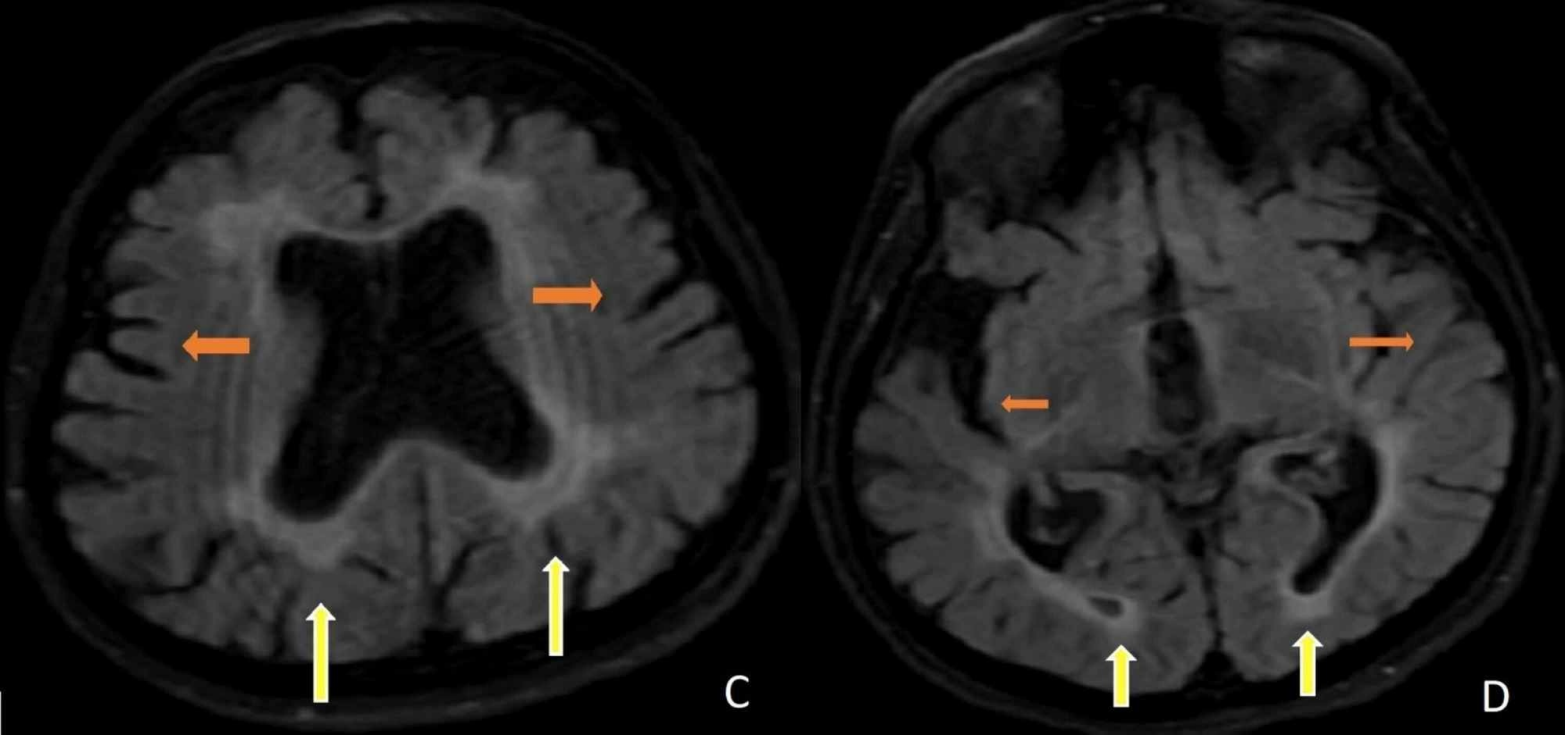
C-D. Axial FLAIR brain MRIs demonstrate diffuse hyperintensities in the periventricular and subcortical white matter (yellow arrows) with cerebral atrophy (orange arrows). FLAIR: fluid-attenuated inversion recovery; MRI: magnetic resonance imaging
